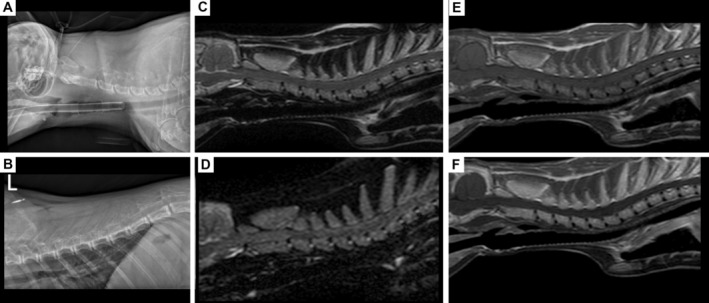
Lateral radiograph of the cervical and thoracic vertebral column showing markedly reduced mineral opacity and cortical thinning in the axial skeleton (A, B). Magnetic resonance imaging of the cervical vertebral column demonstrating the marked hyperintensity of the included skeleton in T2w (C), STIR (D) and T1w (E) sequences. After gadolinium administration, a strong, diffuse contrast enhancement of the bones is demonstrated in the sagittal post‐contrast T1w sequence (F).
